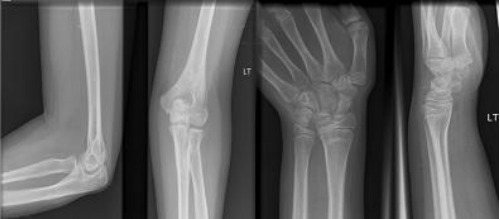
Radiographs of the elbow and wrist after removal of the metalwork.
